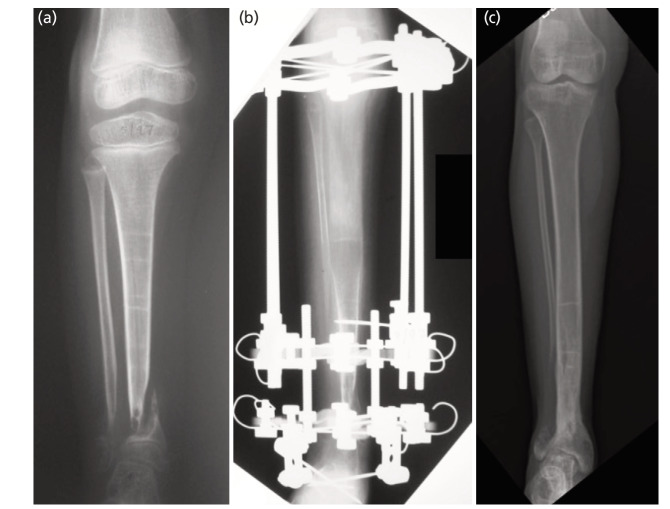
Tibial radiographs of Case 1. Bone union was obtained at age 7 and no refractures. She had mild residual deformity - Johnston Grade I: (a) Congenital pseudoarthrosis of the tibia and fibula at age 6 years. (b) IEF lengthening at age 6 years. (c) Distal tibial valgus and 2cm LLD at age 23 years.
